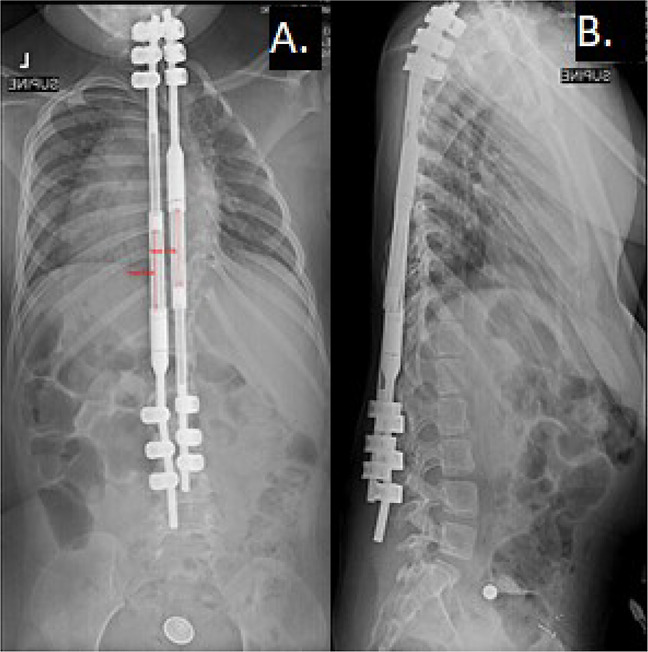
Four-year postoperative radiographic images demonstrating MAGEC rods at maximal distraction. The right rod was maximally distracted to 47.7 mm, and the left rod was maximally distracted to 48.3 mm. A, PA view. B, Lateral view.
